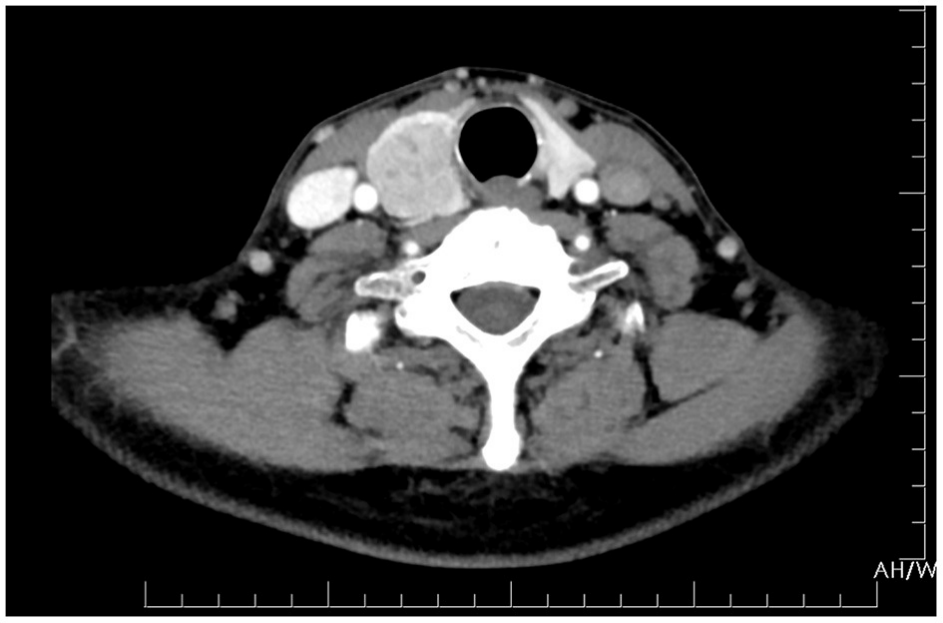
CT of the neck showed a tumor occupying almost the entire right lobe of the thyroid gland.
